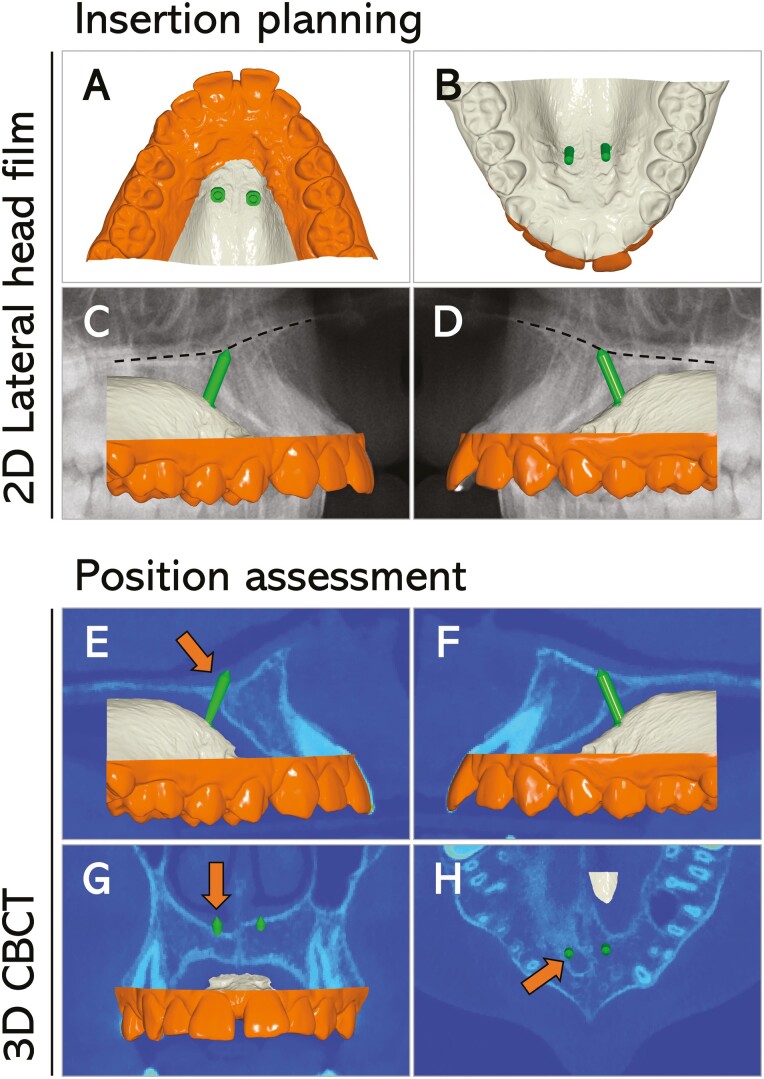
Miniscrew insertion planning using the lateral cephalogram superimposed on the maxillary intraoral scan and the assessment of miniscrews’ positions on the corresponding CBCT image. Miniscrew insertion planning using a subject’s lateral cephalogram superimposed on the maxillary intraoral scan (A–D) and position assessment on the corresponding CBCT image (E–H). Two miniscrews of 9 mm (green) were planned for insertion. Note that the apparent bicortical insertion without any perforations seen on the lateral cephalogram (C, D) was disproved by the assessment on the CBCT image. In detail, the miniscrew on the right side perforated the nasal floor (E, G; orange arrows) and the incisive canal (H, orange arrow). The asymmetry in palatal bone thickness and incisive canal morphology between the right and left sides (G, H) could not be detected on the lateral cephalogram.
